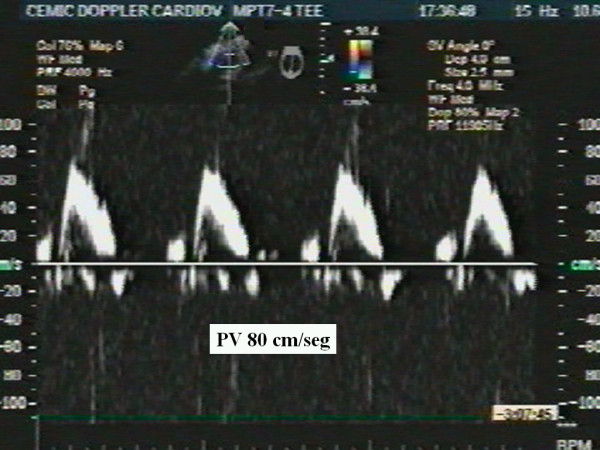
Evaluation with pulse wave Doppler of the right pulmonary artery showing normal flow velocity.
